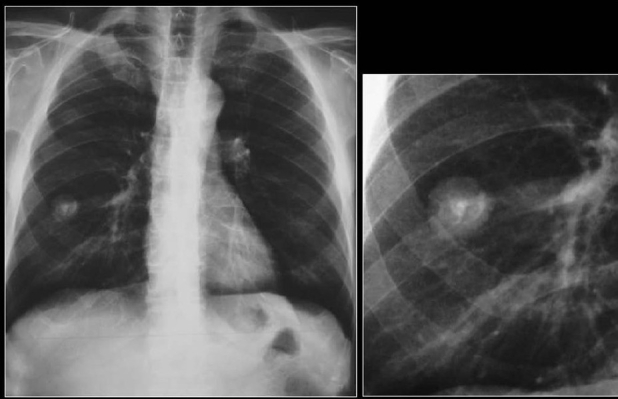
A chest radiograph shows a fairly well-defined PN in the right mid zone associated with a central nidus and a laminated calcification in a pulmonary hamartoma
